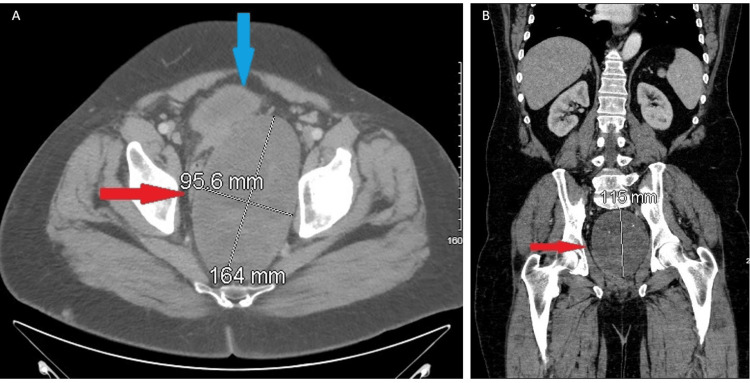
(A-B) CT abdomen/pelvis with contrast. Complex, heterogeneous, encapsulated solid mass (red arrow) within the pelvis measuring up to 16.4 cm. Markedly enlarged, heterogeneous, and infiltrative prostate gland (blue arrow), which obliterates the seminal vesicles and invades the urinary bladder in keeping with advanced prostate carcinoma.
